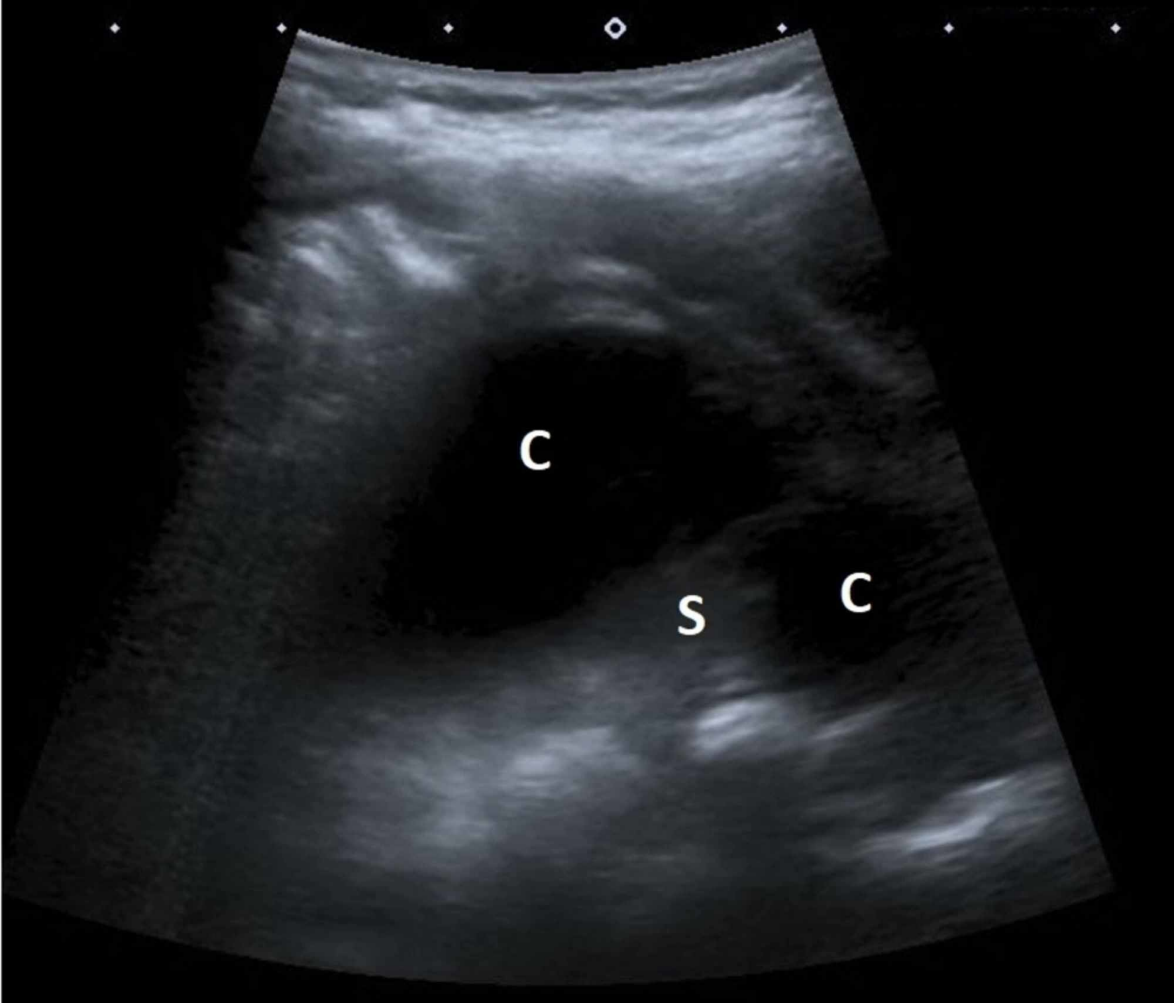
US examination of the neck showing a partly cystic (C) partly solid (S) change. US, ultrasound
